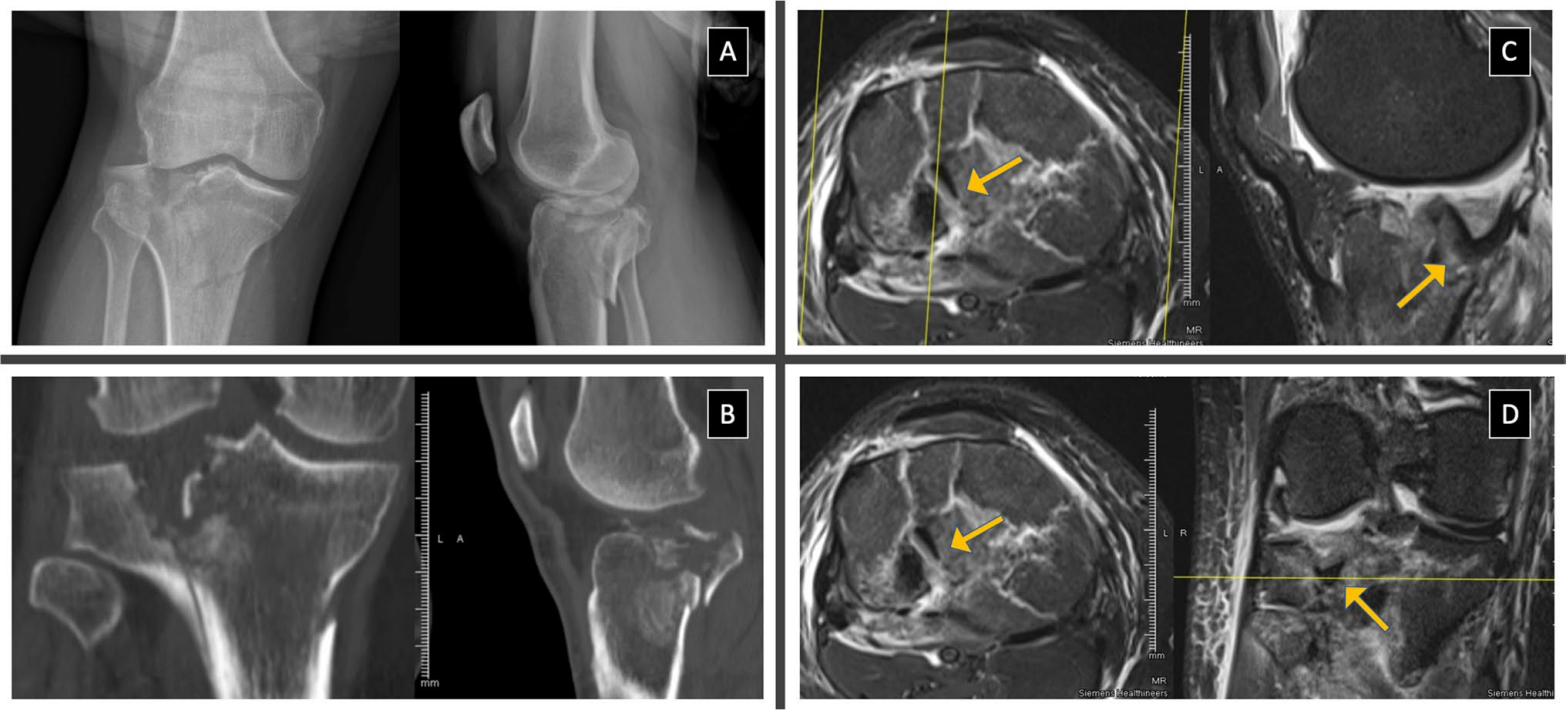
Multifragmentary tibial plateau fracture with postero-lateral depression and displaced lateral meniscus (LM) into the fracture. A anteroposterior (AP) and lateral X-ray views; B, Coronal and sagittal computed tomography (CT) scans; C Axial and sagittal T2-weighted fat-suppressed fast-spin-echo (FSE) sequences; D Axial and coronal T2-weighted fat-suppressed fast-spin-echo (FSE) sequences. The arrow (yellow) indicates the entrapment of the injured LM segment within the postero-lateral tibial plateau fracture
